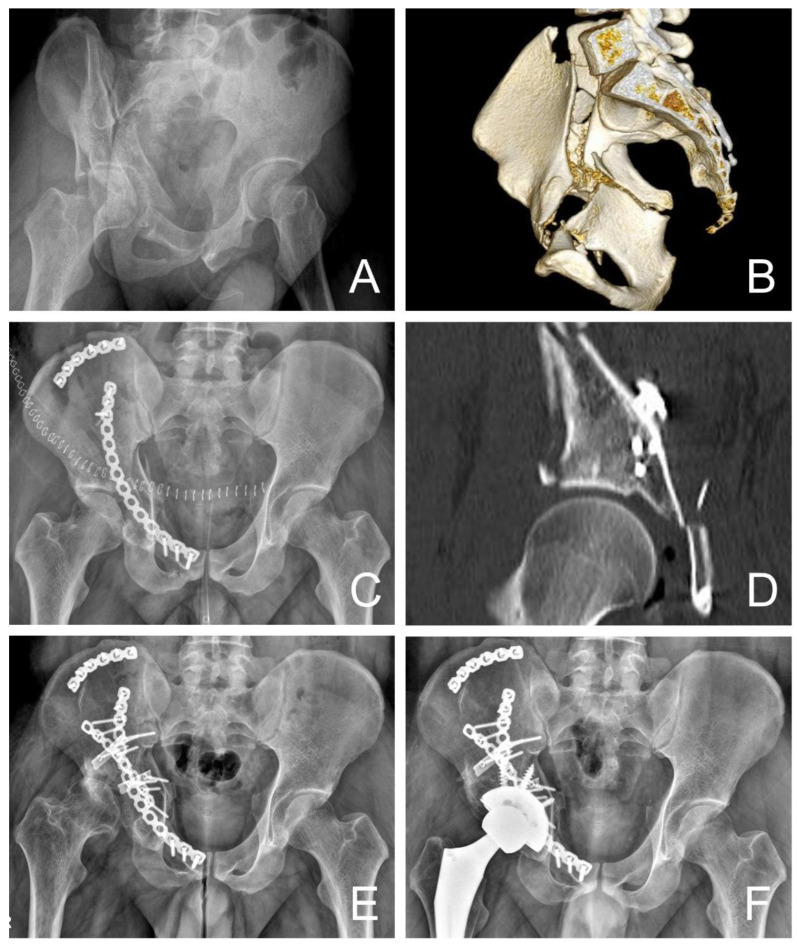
A 27-year-old man underwent indirect fixation with suprapectineal plate. (A) Radiograph shows both-column fracture with 20 mm displacement. (B) Three-dimensional computed tomography (CT) scan shows both-column fracture with quadrilateral plate displacement. (C) Indirect fixation with suprapectineal plate is performed. (D) CT scan shows poor reduction. (E) Radiograph obtained 17 months after surgery shows poor grade with Matta’s outcome grading with joint space narrowing above 50% and severe subchondral sclerosis. (F) Radiograph obtained after conversion of total hip arthroplasty, 17 months after initial surgery. CT = computed tomography.
